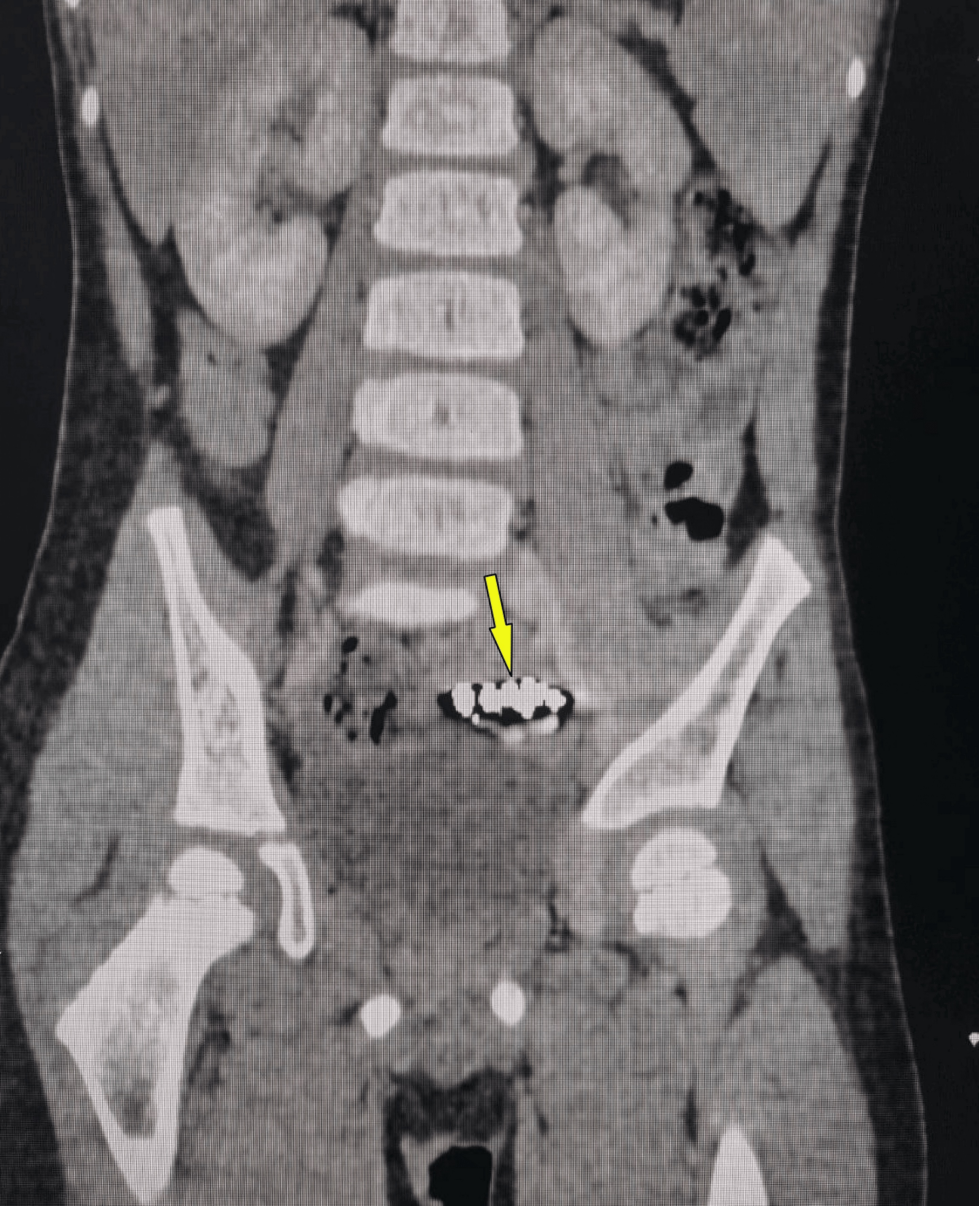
CT abdomen demonstrated a large, irregular FB (arrow) within the distal ileum FB: foreign body
